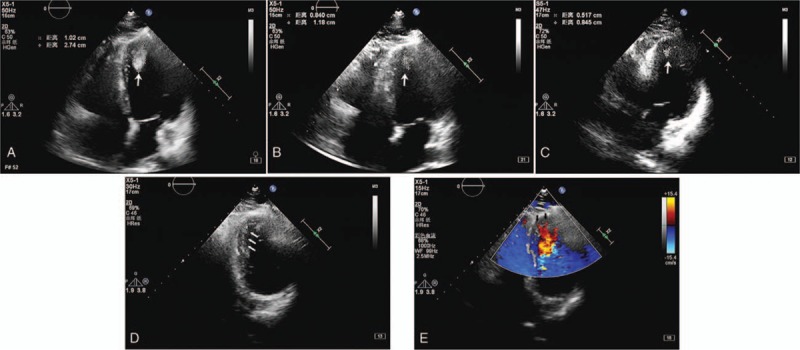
Serial echocardiographic images in the identification of a left ventricular thrombosis-like mass. (A) Identification of a thrombus at the left ventricular apex during the initial echo examination, leading to the initialization of rivaroxaban (10 mg daily) treatment. Crossed lines surrounded the thrombus-like mass, which is also indicated by an arrow. (B) After 15 days of treatment, the size of the thrombus was reduced. (C) At 45 days of anticoagulation therapy, the size of the thrombus was further reduced. (D) The thrombus had diminished after 3 months, revealing suspected increased intratrabacular recesses of the heart (blue arrows). (E) Doppler imaging revealed a “flushed” pattern of blood flow into the myocardium.
